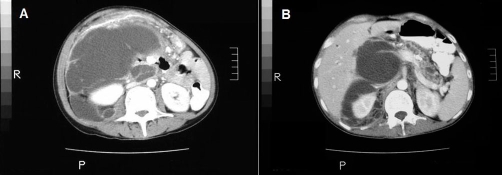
(A and B) Abdominal CT scan of the patient (A, B): (A) Complex cystic retroperitoneal mass 20 cm in diameter, extending from liver to pelvis; (B) Dilatation of the intra and extrahepatic bile ducts, atrophy and parenchymal calsifications of the pancreas and dilatation of the Wirsung’s duct.
